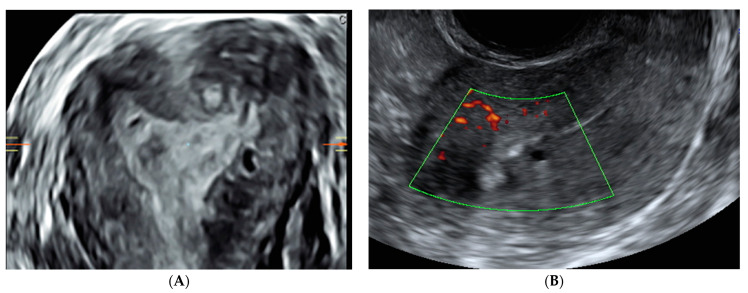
(A) Transvaginal three-dimensional ultrasound images of a uterus affected by subendometrial hyperechogenic lines and buds infiltrating the JZ (3D TVS). (B) Transvaginal two-dimensional ultrasound images of a uterus affected by focal adenomyosis of the JZ with myometrial cysts and hyperechogenic islands (2D TVS).
